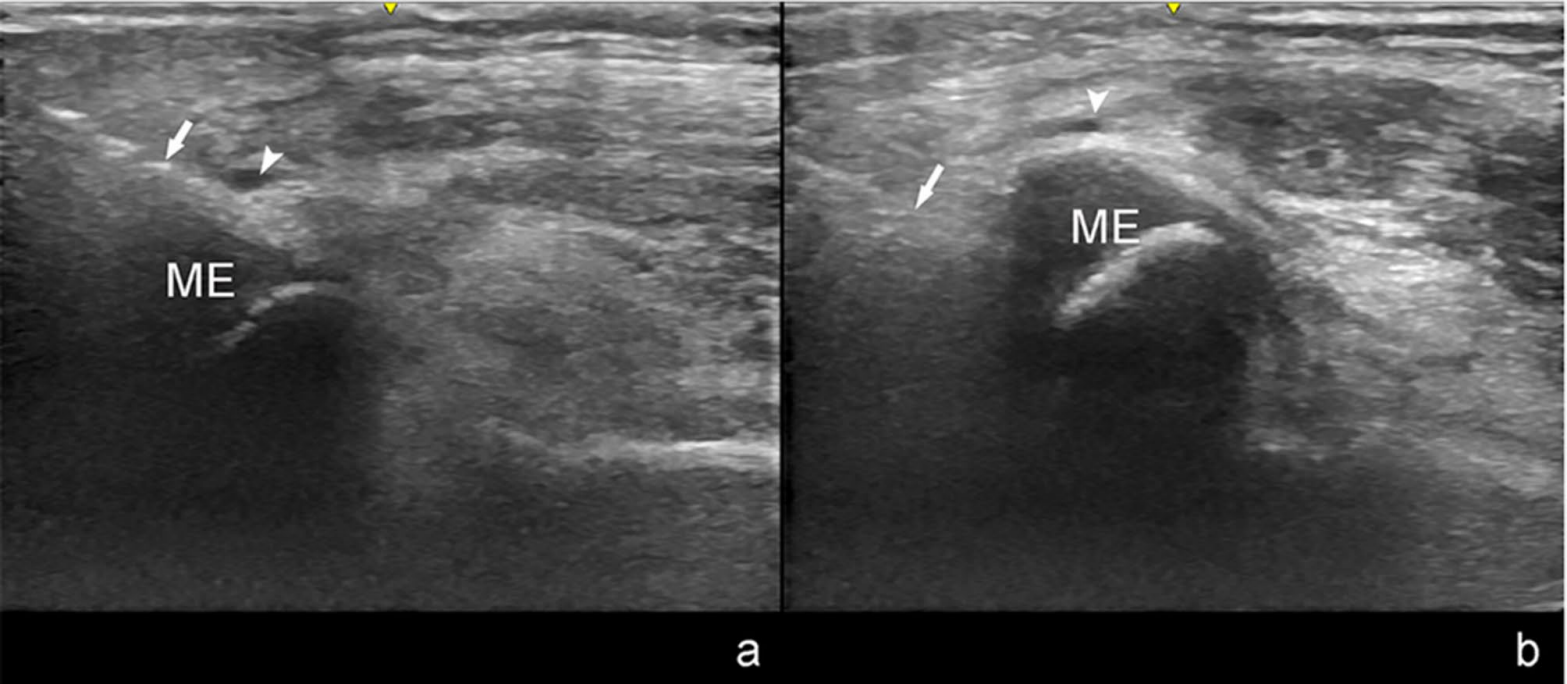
A 59-month-old boy, (a) Oblique section ultrasound showed the proximity of the inserted medial pin to the ulnar nerve. b The distance between the ulnar nerve and the medial pin could be seen after repeated pin insertion. ME: medial epicondyle of humerus; arrowhead: ulnar nerve; arrow: the medial pin
